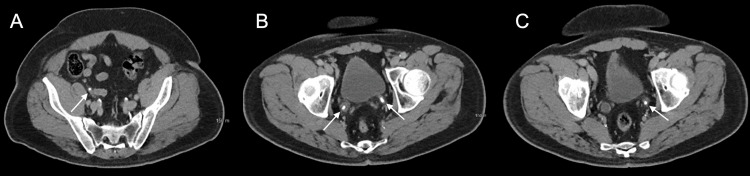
Axial images from the patient's CT abdomen and pelvis revealed four subcentimeter stones. The stones (white arrows) are seen in the right mid-ureter (A) and bilateral ureterovesicular junctions (B,C), with right greater than left distal ureteral dilation (B,C).
